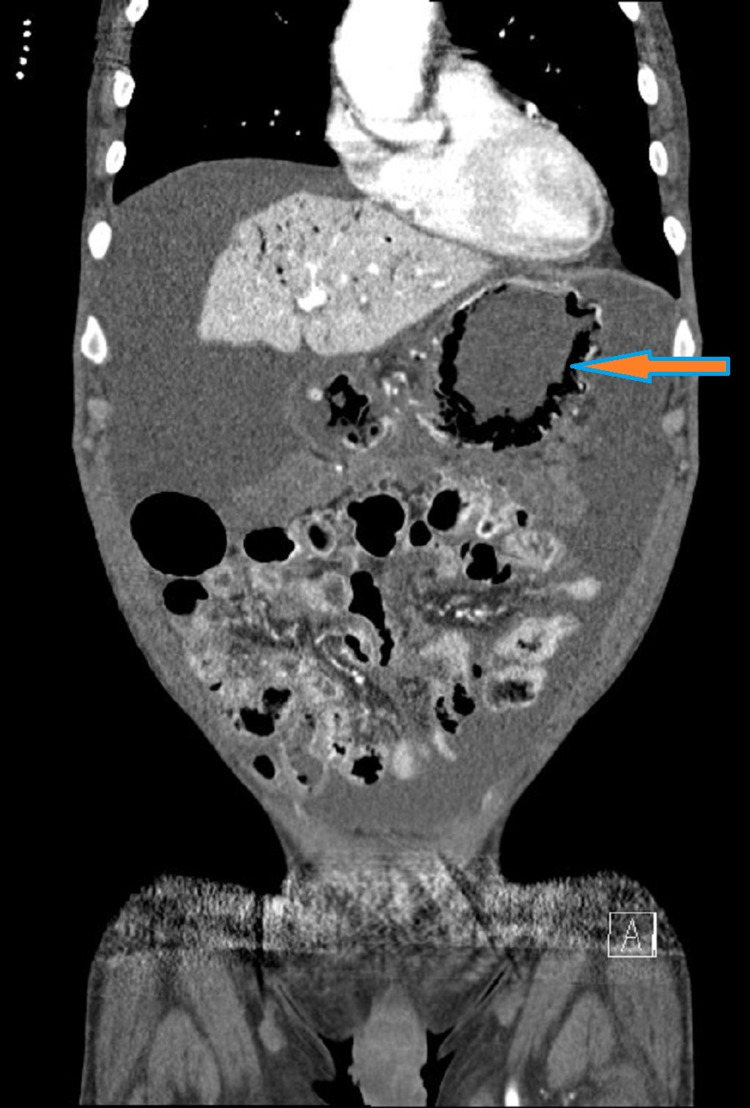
Coronal sectional view of the CT scan of the abdomen showing gas within the wall of the stomach. Arrow demonstrating intra-mural gastric air.
